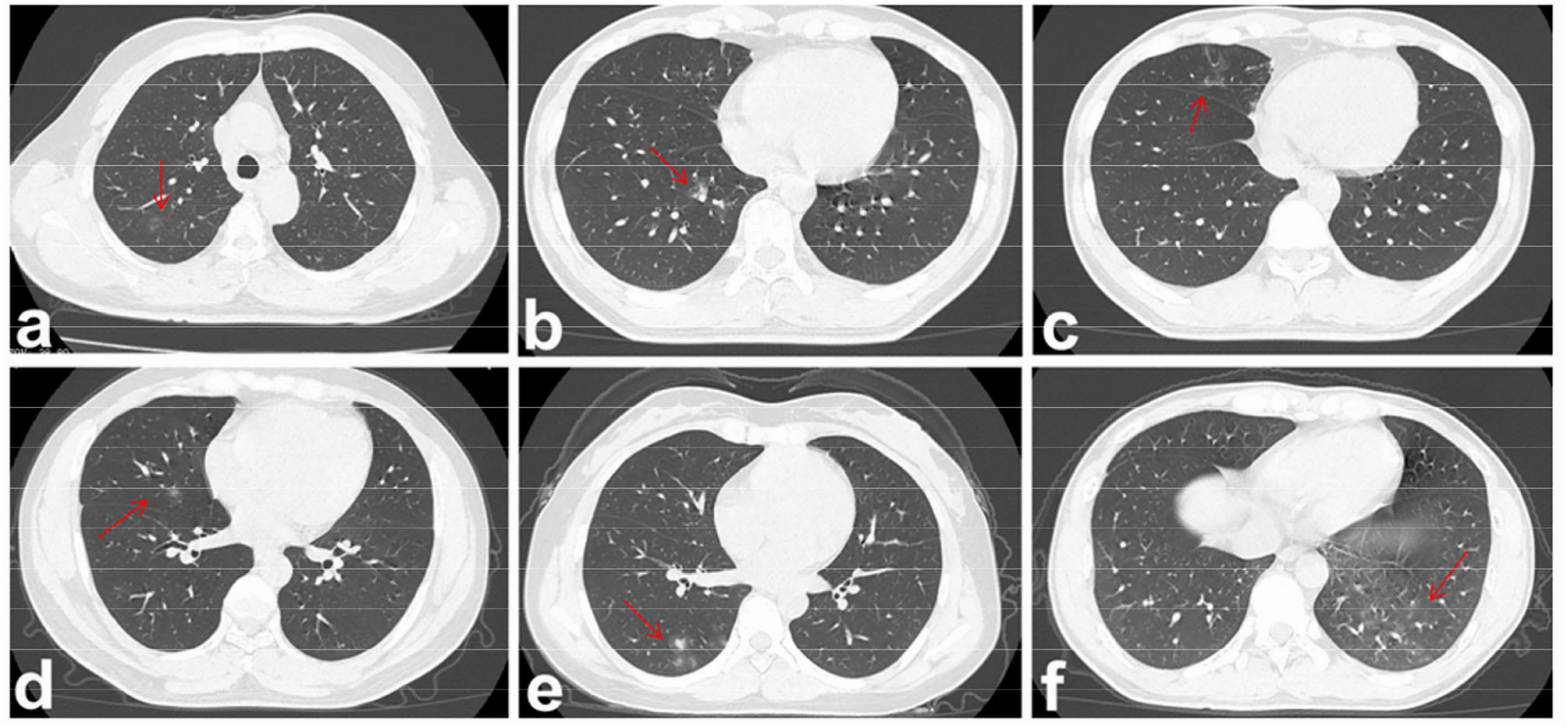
Viral pneumonia of pulmonary CT imaging in the five patients infected with Omicron variant. The lesions were marked by red arrows in panels (a–f). (a) Patient 1: GGO in the right upper lobe of a 38-year-old woman. She presented with fever and sore throat. (b) and (c) Patient 2: GGO in the right lower and middle lobe of a 27-year-old man, respectively. He presented only with low degree of fever. (d) Patient 3: GGO in the right middle lobe of a 28-year-old man. He was asymptomatic. (e) Patient 4: GGO in the right lower lobe of a 28-year-old woman. She presented with body pain and fatigue. (f) Patient 5: GGO in the left lower lobe of a 29-year-old man. He presented with fever and sore throat.
